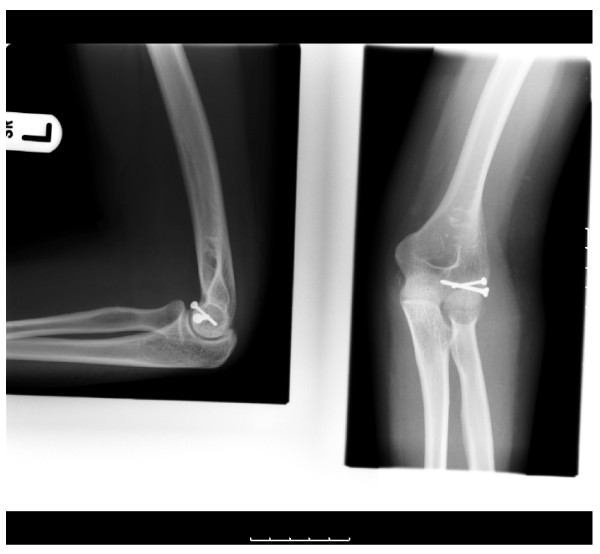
Radiograph after fixation.
